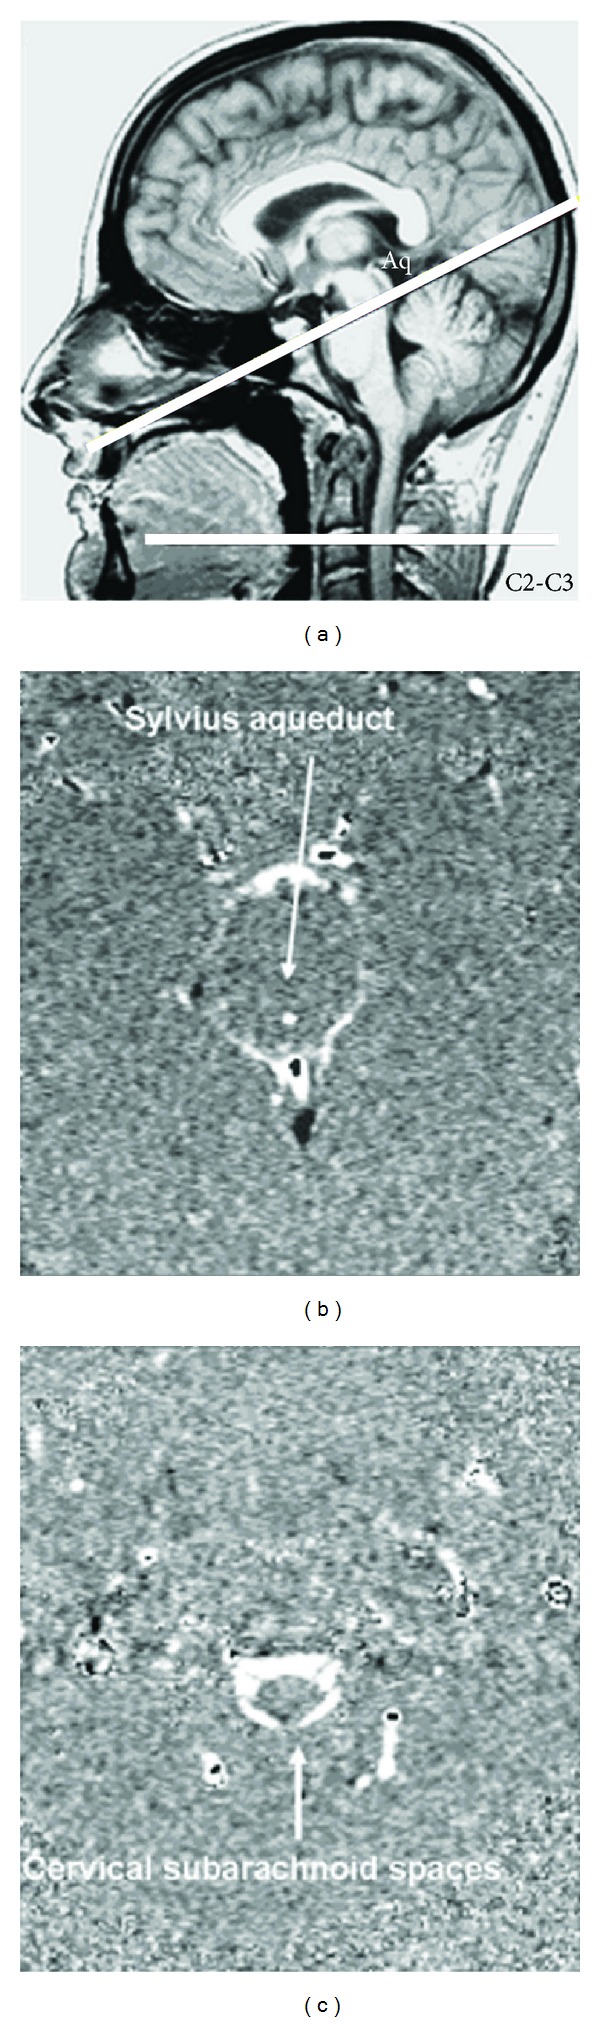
Data acquisition by phase-contrast magnetic resonance imaging: the selected acquisition planes were perpendicular to the presumed flow direction. Sections through the aqueduct of Sylvius and the C2-C3 subarachnoid space (C2-C3) were used for cerebrospinal fluid flow measurement (a). Examples of phase contrast images at the Sylvian aqueduct and the cervical subarachnoid spaces are shown in images (b) and (c).
